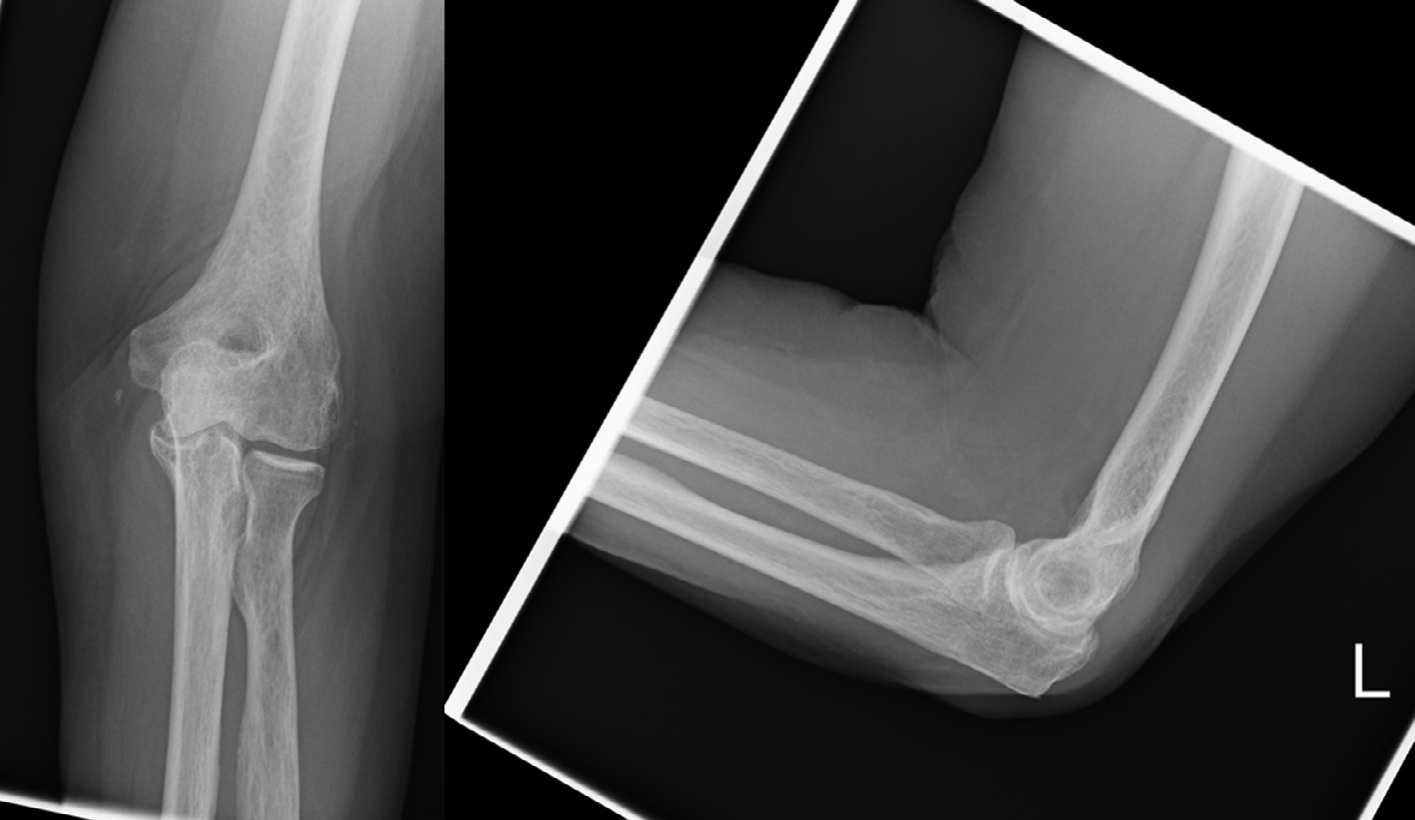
Elbow AP and lateral radiographs two years post reconstruction.
